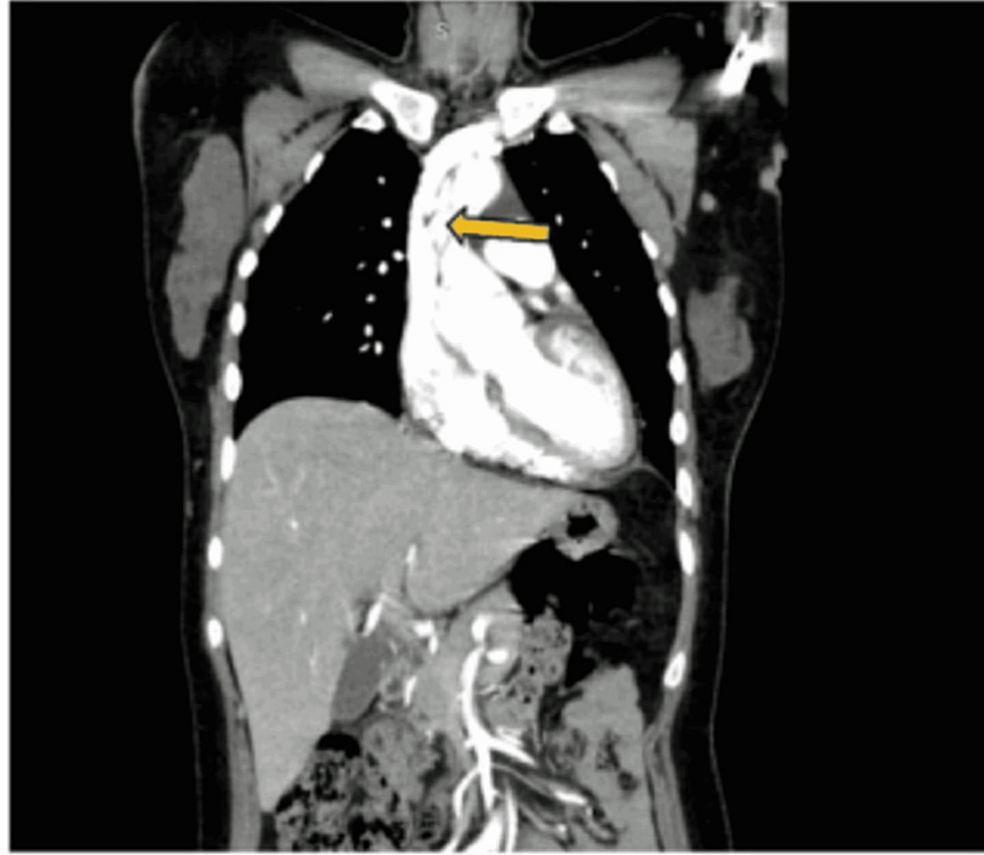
CT aortogram showing Stanford type A aortic dissection (arrow) (coronal view)
